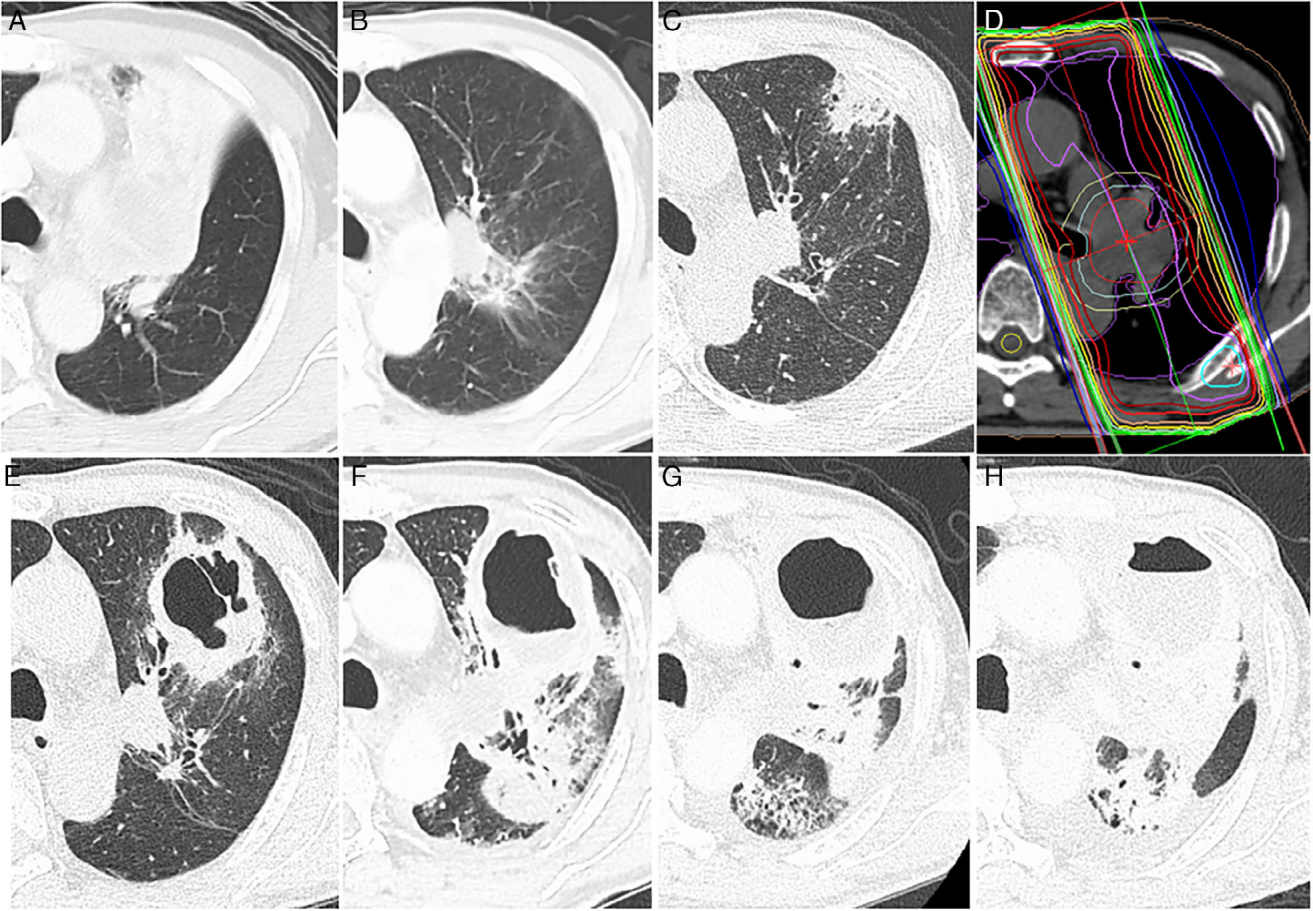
Computed tomography (CT) scan taken at diagnosis of lung cancer showing a hilar tumour causing atelectasis of the left upper lobe (A). CT scan taken after completion of chemoradiotherapy revealing marked decrease in the primary lesion as well as resolution of the atelectasis (B). CT scan on readmission showing lung infiltrate in the left upper lobe (C). CT imaging for radiotherapy planning indicating that the lung infiltrate was outside the radiation field (D). CT scans taken on day 14 (E), day 33 (F), day 49 (G), and day 82 (H) of readmission showing development of the cavitary lesion.
